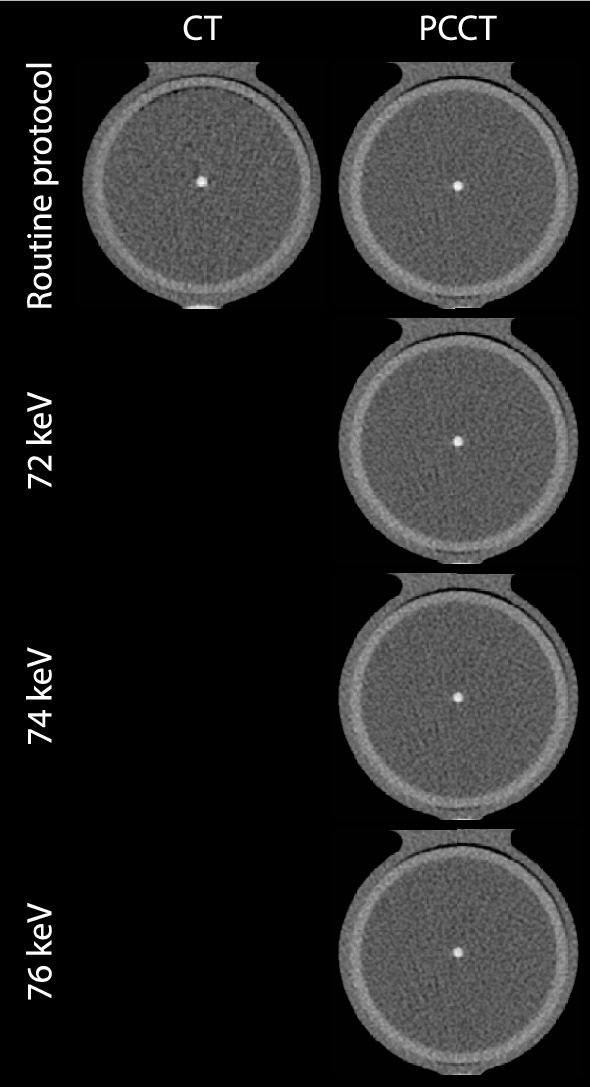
Representative images for the medium density CAC for both CT (left) and PCCT (right) at 60–75 bpm. The upper row represents the routine clinical protocol, with a monoE level of 70 keV for PCCT. Increased monoE levels images are shown in the lower rows
